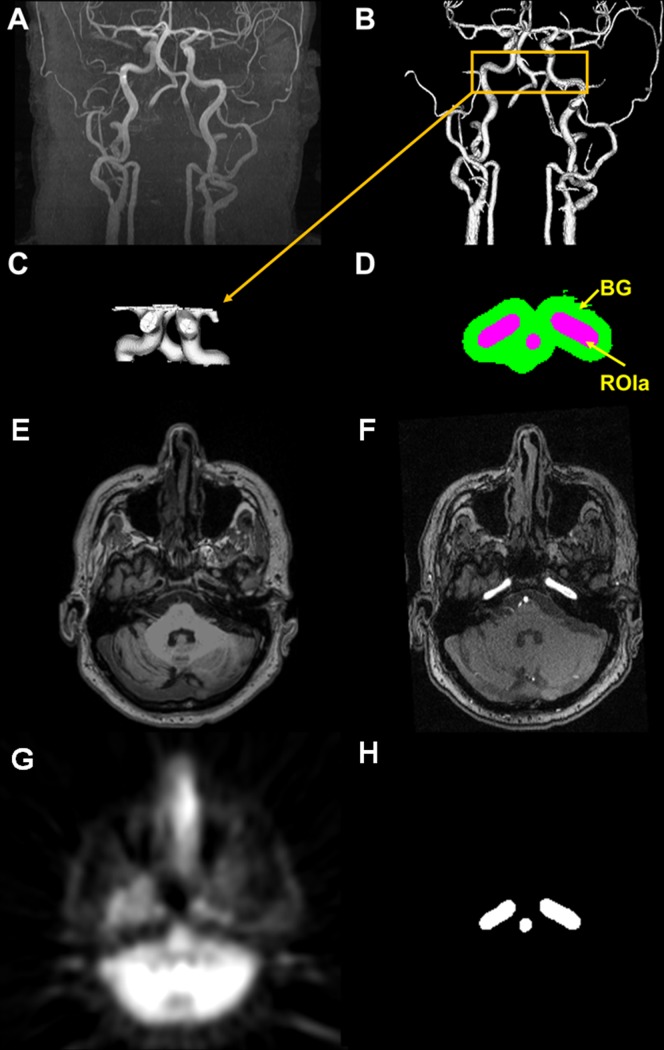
Three dimensional rendering of time-of-flight MRA (A), segmented arterial tree (B), the arterial region-of-interest (ROIa) (C), and one example slice of the arterial ROI (ROIa) and the background region-of-interest (BG) (D). Example slice of coregistered MRI (E), MRA (F), PET(G), and ROIa (H).
